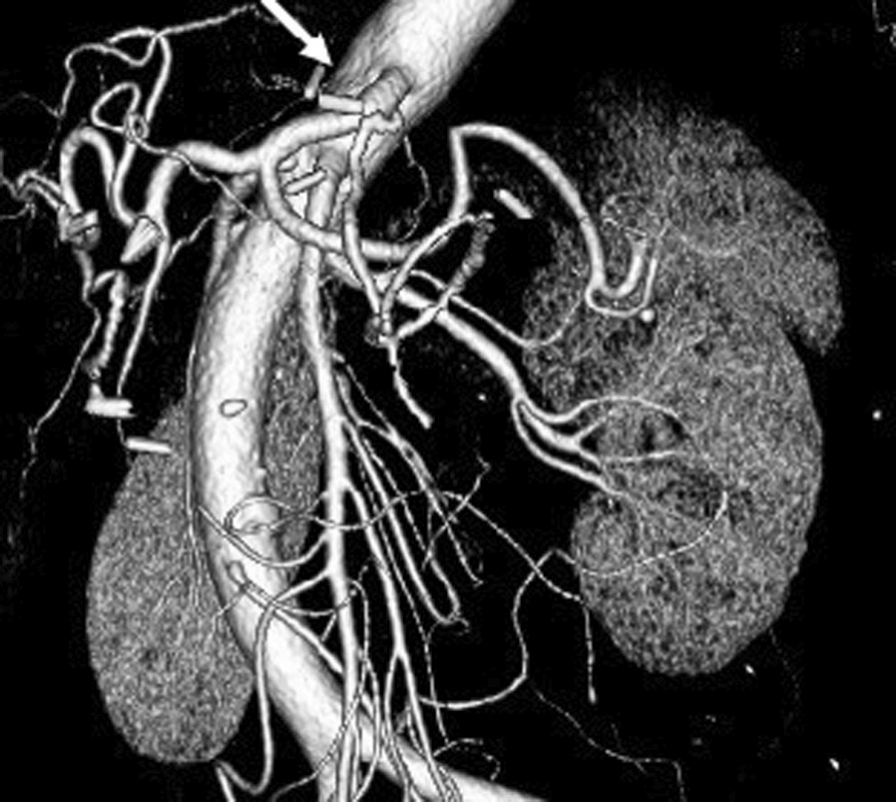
3-D CT angiography was performed about 2 months after the operation, and it was confirmed that the stenosis of the celiac artery due to MALS had been resolved (allowed)
